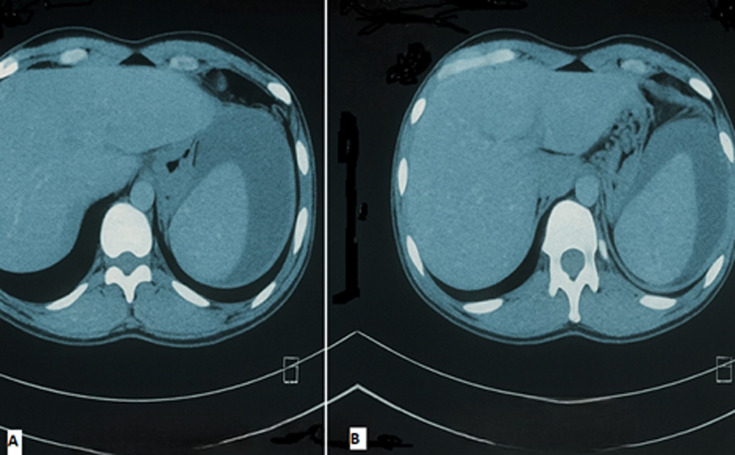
A, B) computed tomography scan of the abdomen with IV contrast showing an enlarged spleen with subcapsular splenic haematoma compressing the splenic parenchyma, consistent with spontaneous splenic rupture
